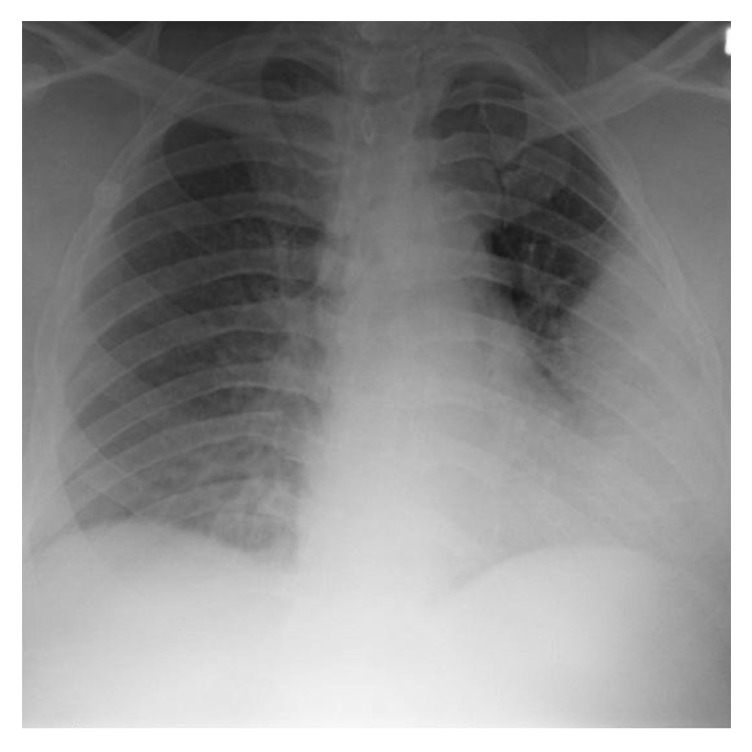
Chest X-ray with homogenous consolidation in the left lower lung field.
